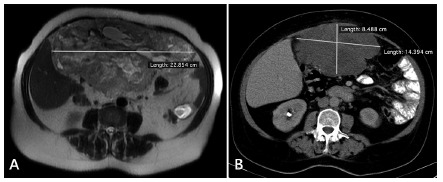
A) Magnetic resonance imaging of the abdomen before neoadjuvant therapy showing a tumor with 22,8 cm in its greater diameter; B) computed tomographic image after neoadjuvant therapy.
